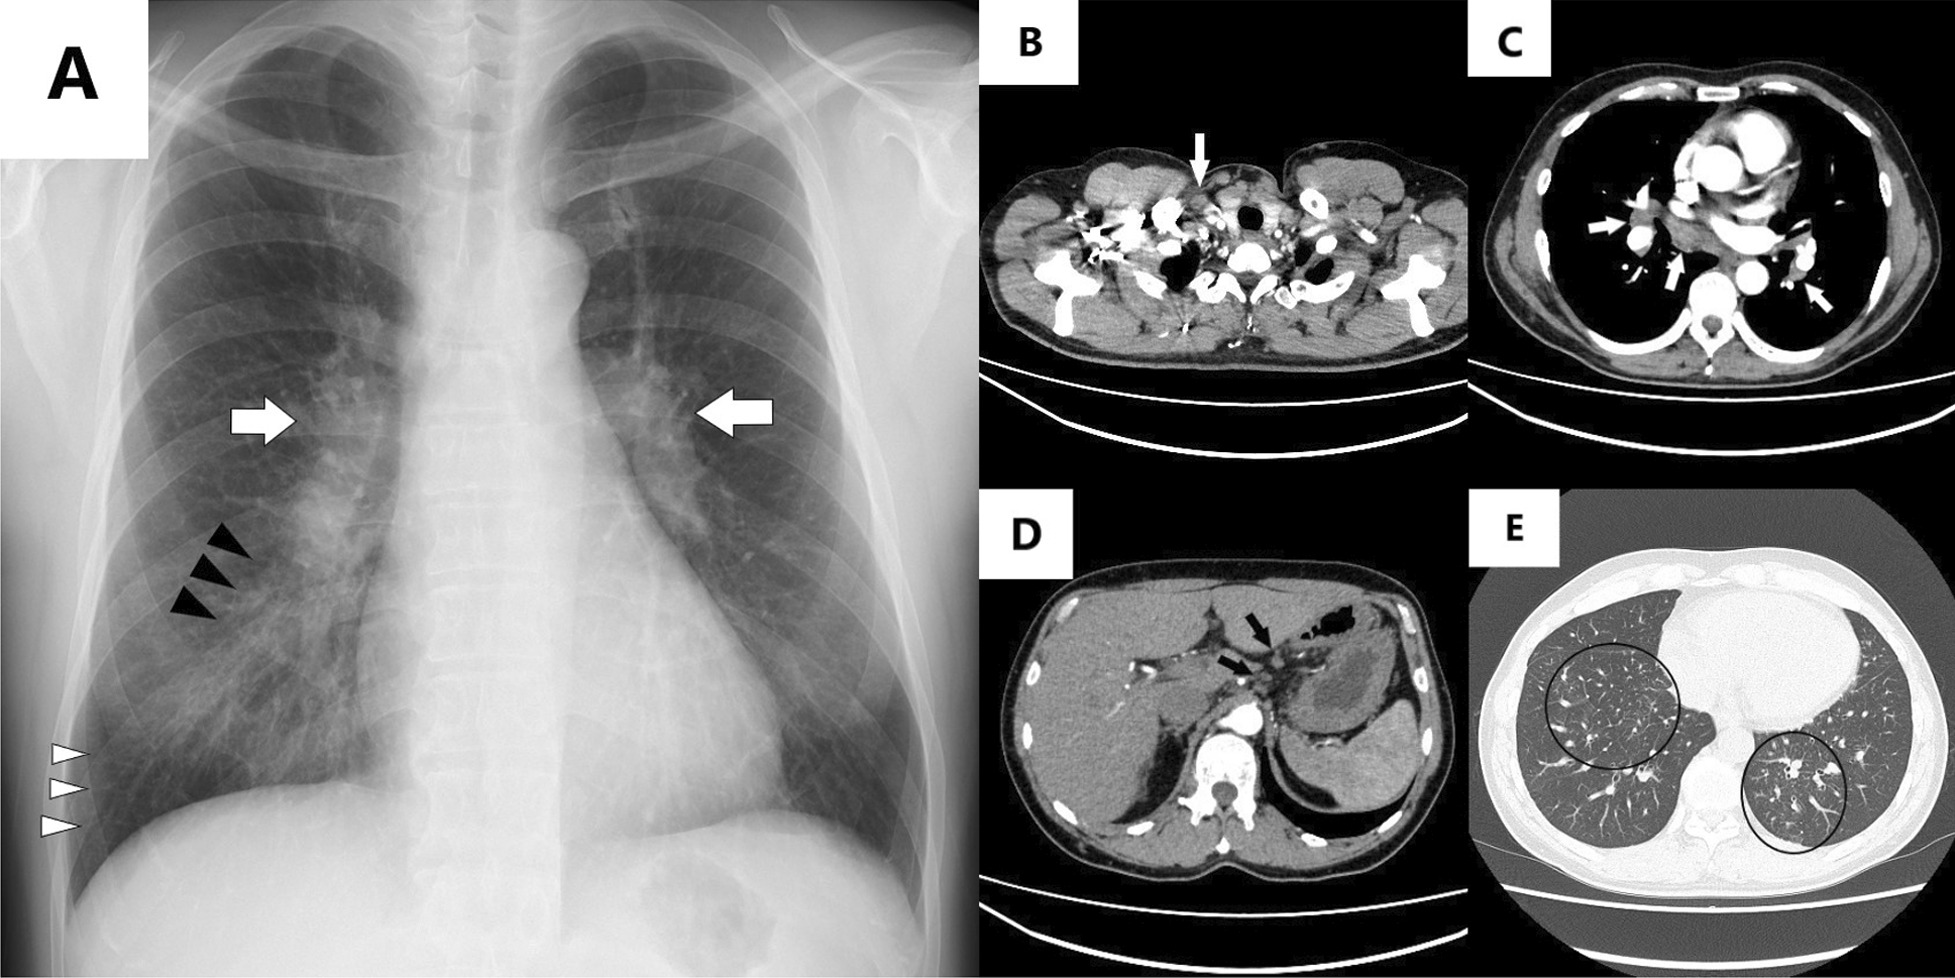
Radiographic findings on admission. A Chest radiography shows bilateral hilar lymphadenopathy (white arrows), ground-glass opacities (black arrows), and Kerley B lines (white arrowheads) in the right lung. Enhanced computed tomography shows B right cervical lymphadenopathy (white arrow), C hilar/mediastinal lymphadenopathy (white arrows), and D upper abdominal lymphadenopathy (black arrows). E Septal thickening and ground-glass opacities are observed in the lower lung lobes (black circles)
